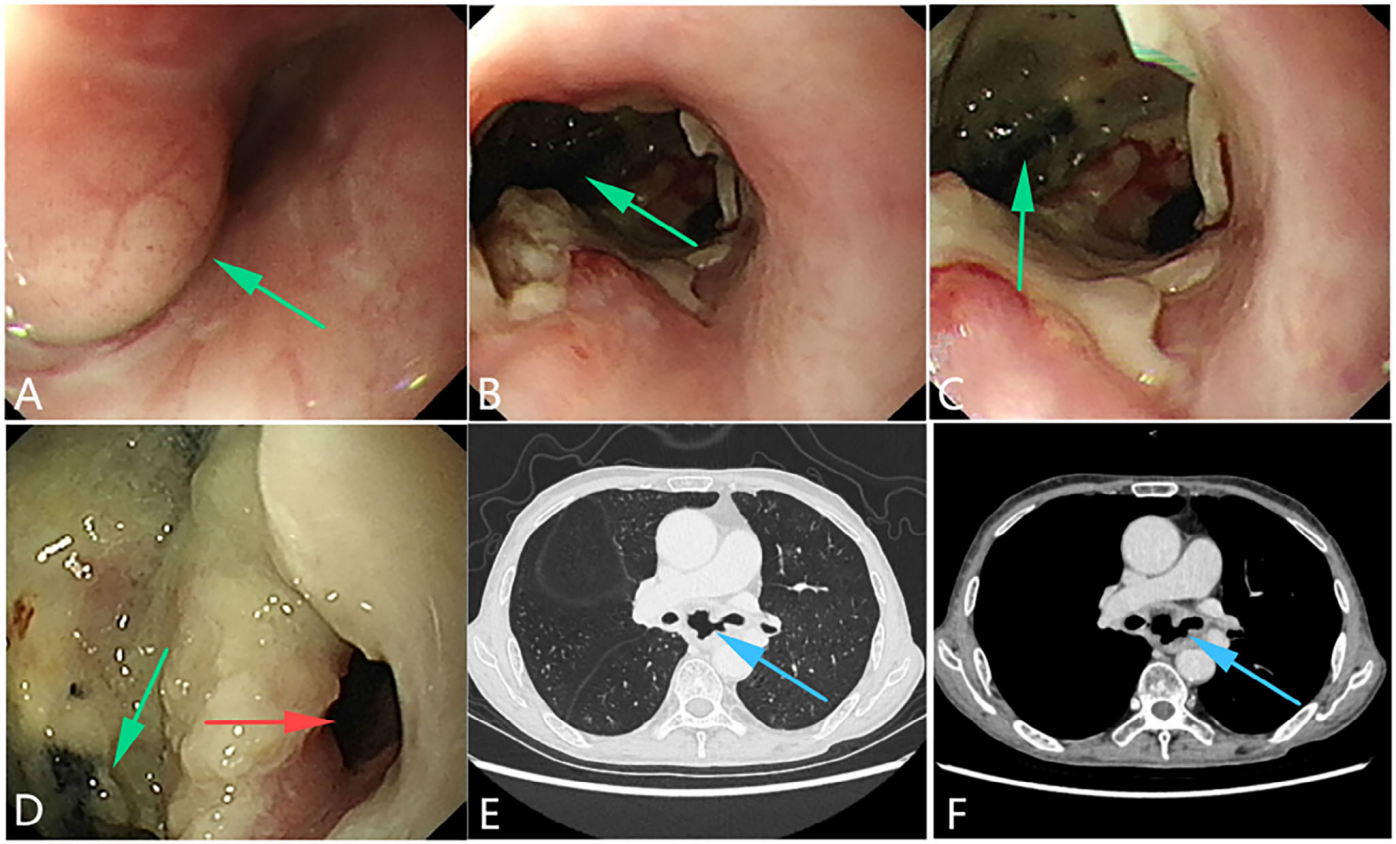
Tracheoesophageal fistula formed after radiation therapy for malignant lung tumors. A) Gastroscopy showed that a malignant lung tumor was compressing the esophageal cavity, causing it to narrow; the surface mucosa was intact and had not yet been broken through. B–D) The patient underwent radiation therapy, and a follow‐up gastroscopy 2.5 months later revealed an esophageal fistula with an extensive white coating attached to the surface. The green arrow represents the fistula, and the red arrow represents the esophageal cavity. E, F) A CT scan can reveal the pathway between the esophagus and trachea. (Original work created by the authors).
